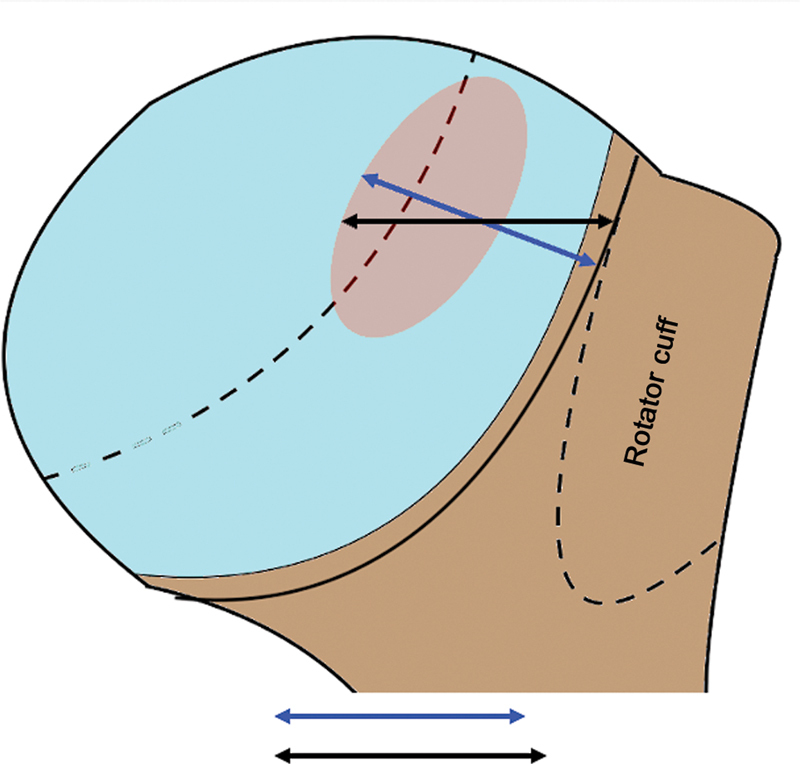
Computed tomography (CT) scans showing the HS lesion. Two-dimensional images in the axial, sagittal, and coronal sections. Three-dimensional images in the lateral and posterior views.
